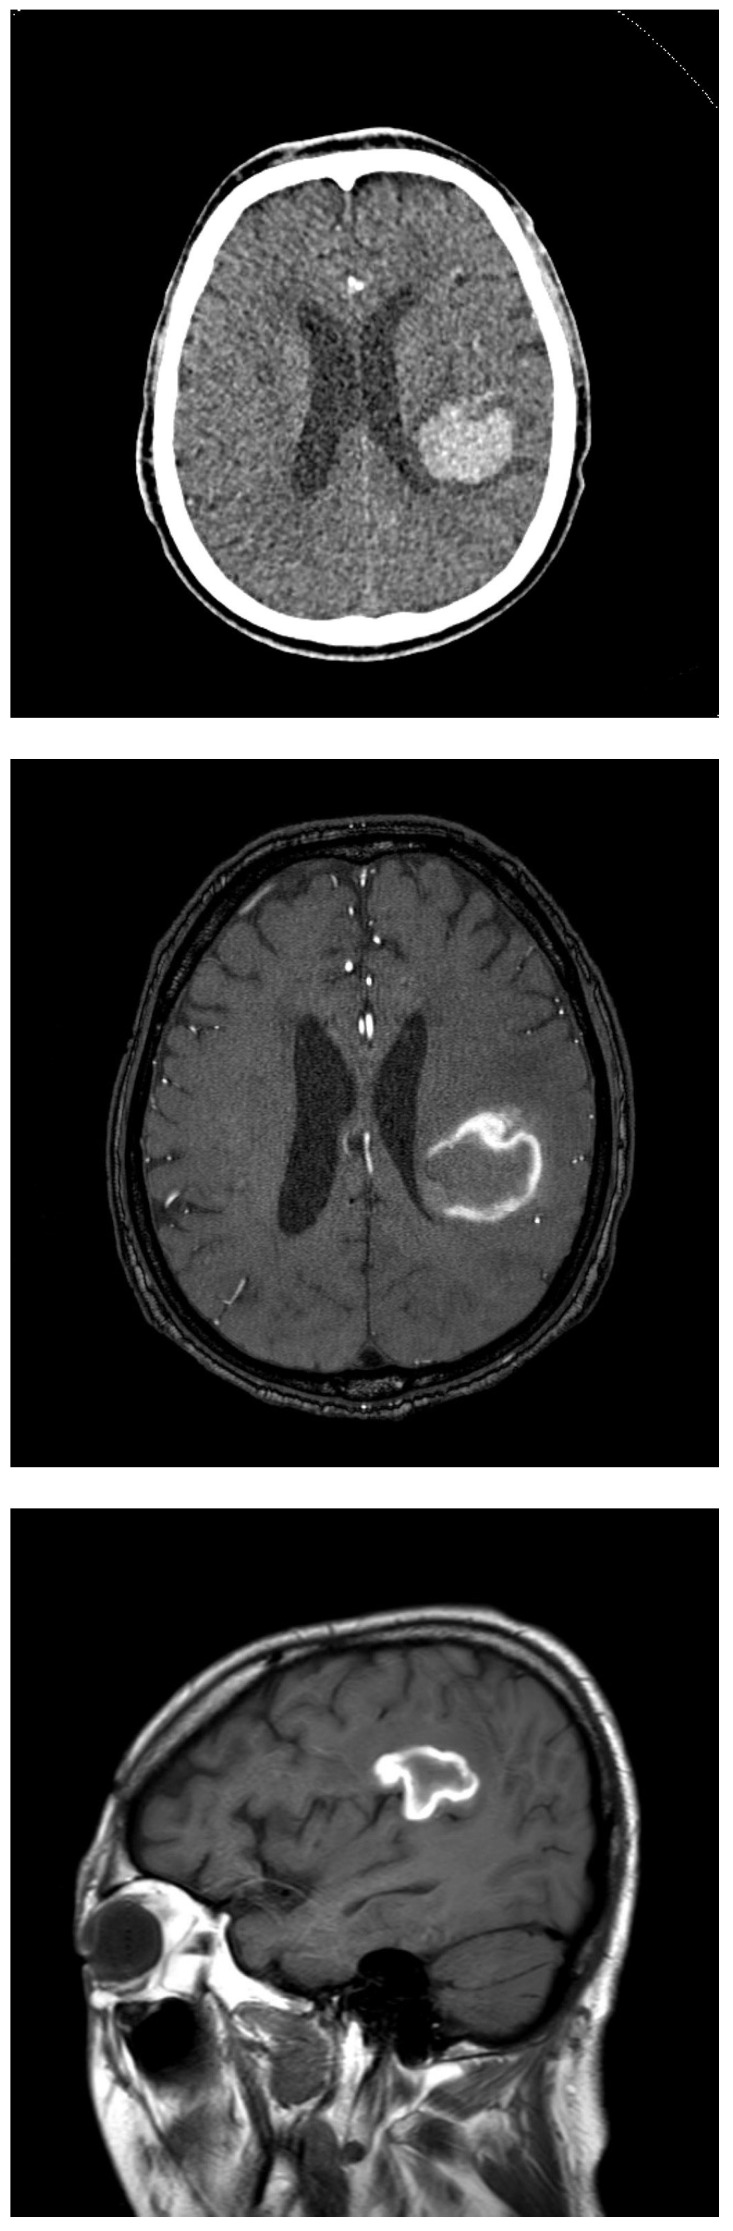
Brain CT and MRI showed findings consistent with acute infarction involving the left frontal lobe and left basal ganglia.
